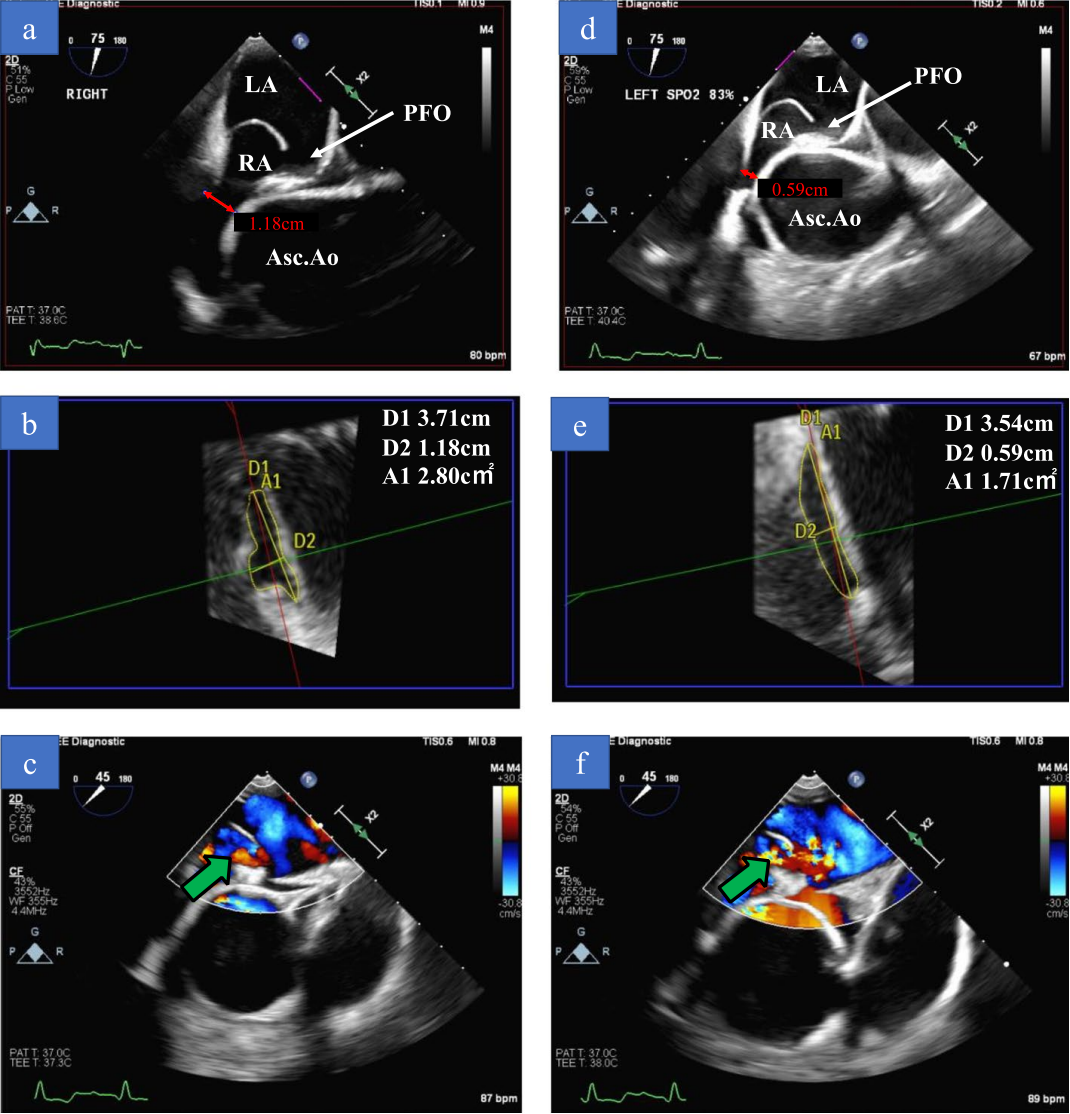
a–c RLDP. d–f Left lateral decubitus position. a and d TEE shows the elongated and dilated ascending aorta causing greater compression of the RA in the LLDP than in the RLDP. b and e Transaxial plane of the RA by TEE showing greater compression of the RA in the LLDP (area 1.71 cm^2^) than in the RLDP (2.80 cm^2^). c and d Shunt flow is enhanced by postural changes from the RLDP to the LLDP. Asc. Ao, ascending aorta; LA, left atrium; RA, right atrium; PFO, patent foramen ovale; RLDP, right lateral decubitus position; TEE, transesophageal echocardiography
